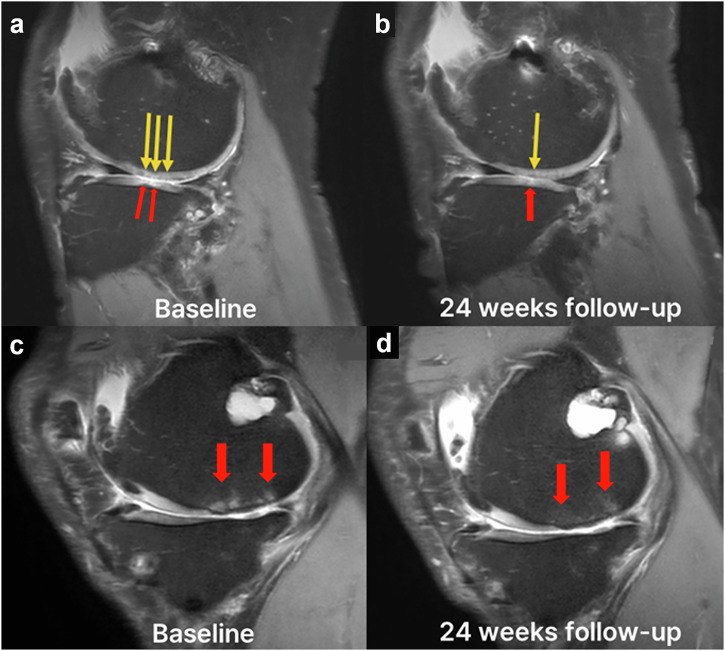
MRI assessment of cartilage repair and subchondral bone changes at baseline and 24 weeks post-OSCA administration. a Sagittal MRI at baseline shows cartilage defects in the lateral femoral condyle (yellow arrow) and lateral tibial plateau (red arrow). b At 24 weeks, MRI revealed increased cartilage coverage in the lateral femoral condyle (yellow arrow) and lateral tibial plateau (red arrow) with surface smoothening. c Baseline sagittal MRI images show subchondral bone marrow edema (red arrows) in the medial femoral condyle. d At 24 weeks, subchondral bone marrow integrity improved (red arrow).
